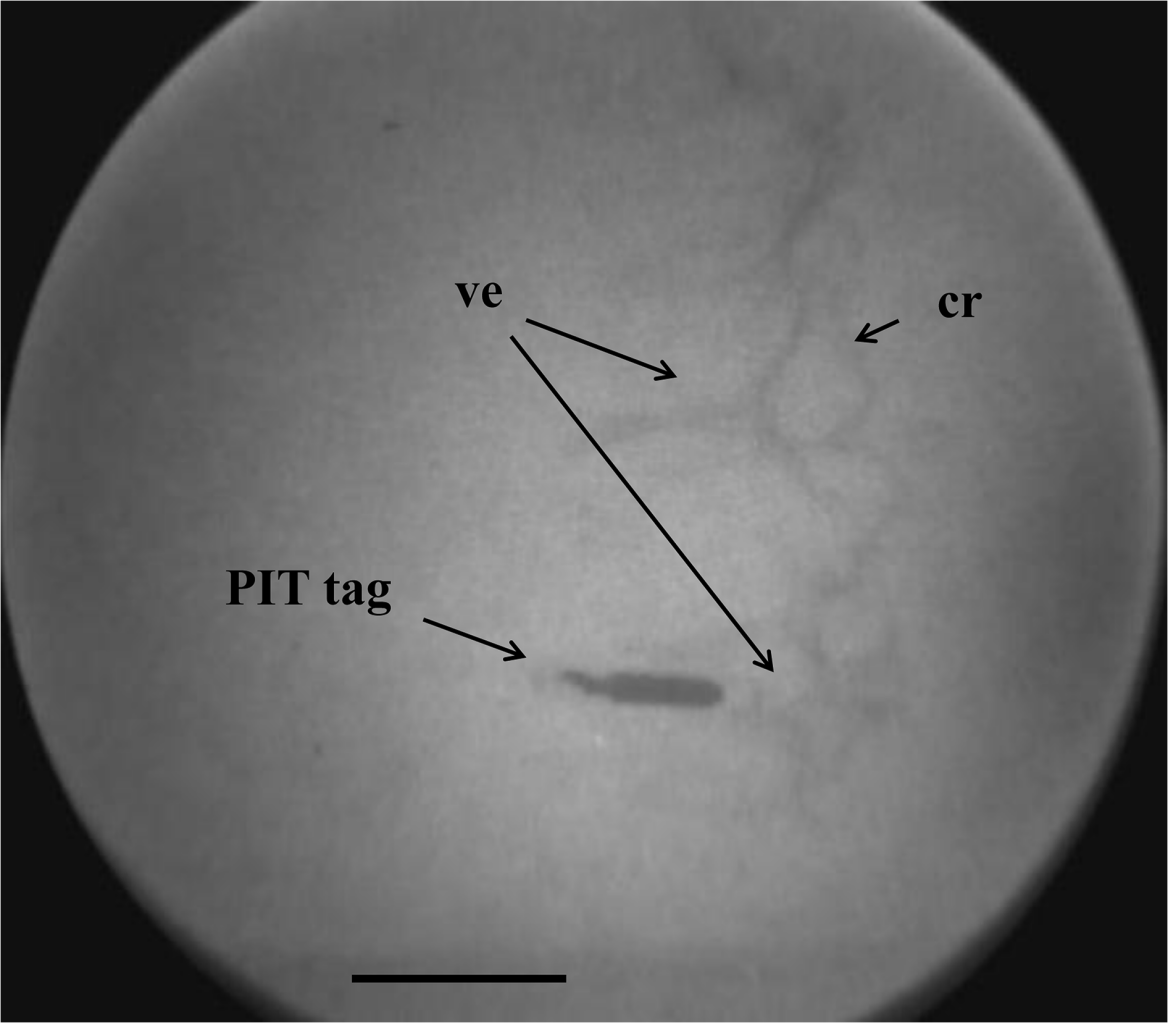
X-ray photograph of sea cucumber tagged in the aquapharyngeal bulb. PIT tags successfully implanted in the aquapharyngeal bulb through a deployed tentacle lodged themselves in one of the tentacle vesicles (ve) close to the calcareous ring (cr). Scale bars represent 2 cm.
